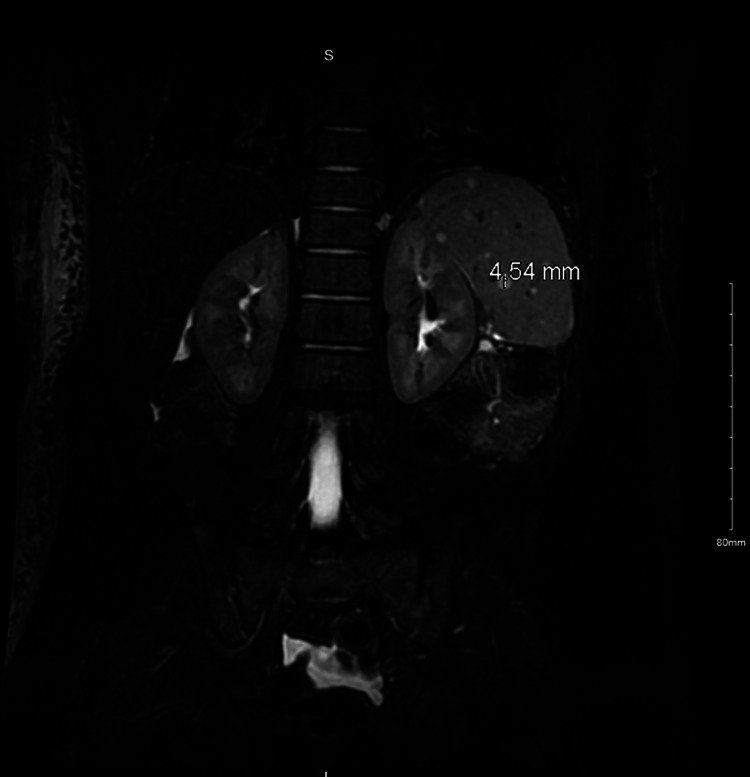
MRI identifying splenic lesions (arrows removed for better visualization) of a 2-year-old with right upper extremity lymphedema
